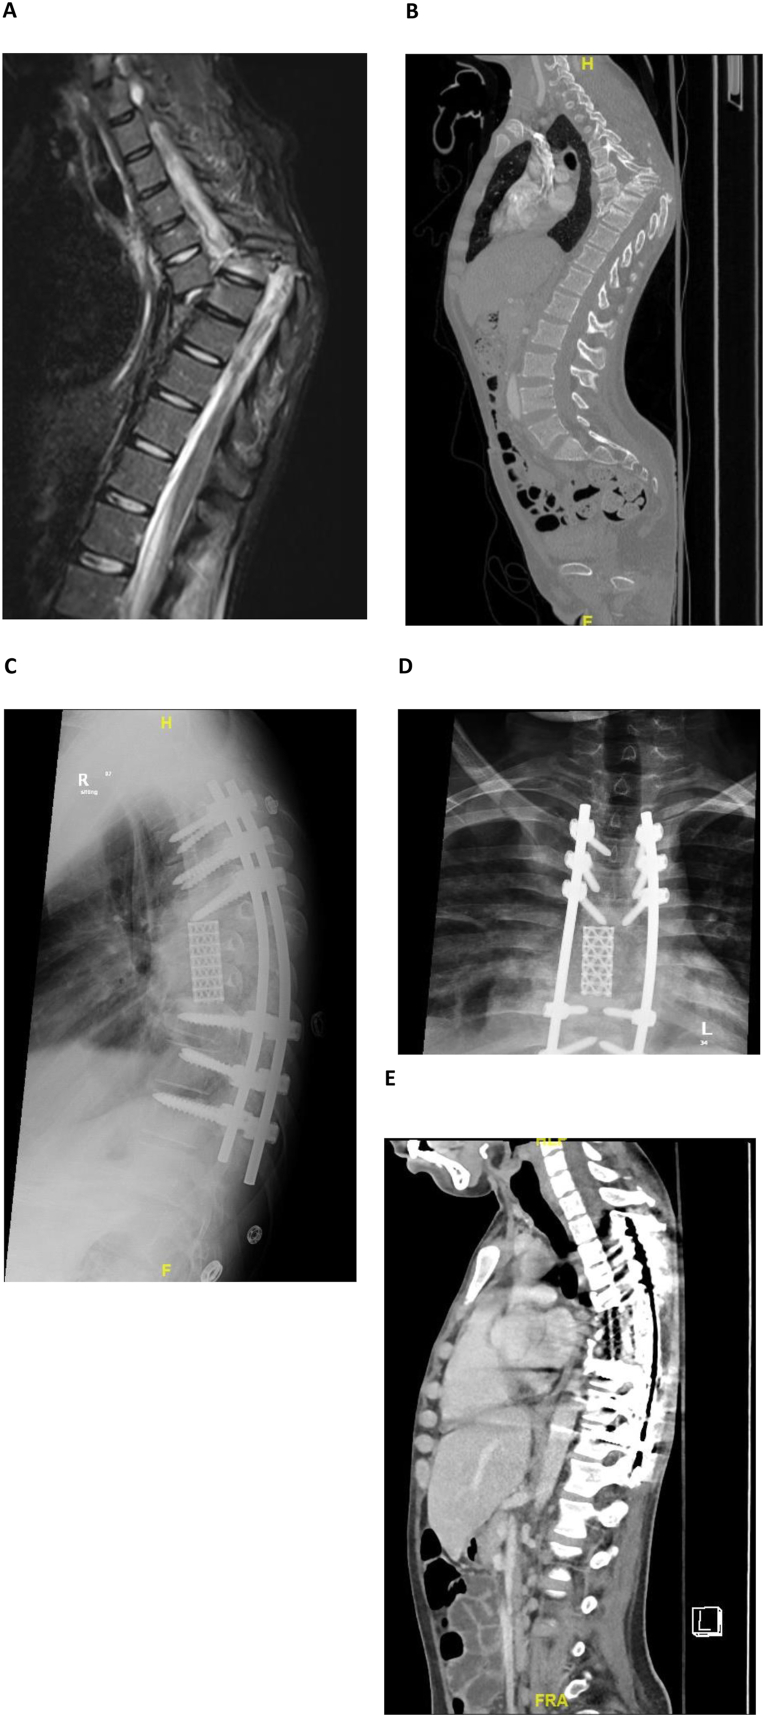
Young patient with neglected T6/7 fracture dislocation with severe kyphotic deformity and overlap (A, B) MRI and CT images showing the severity of the spinal cord injury and spinal column. (C, D, E) X-ray and CT postoperative images showing reconstruction surgery with three levels of corpectomy, multiple levels of fixation with pedicle screws, and reconstruction with a mesh cage.
